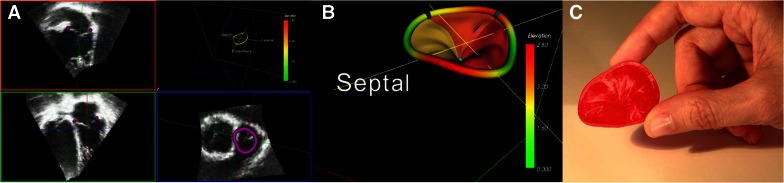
Three-dimensional printing of the tricuspid valve. From three-dimensional transthoracic echocardiographic data of the tricuspid valve, coordinates of the leaflets and annulus are demarcated (A) and imported to dedicated software to create the model (B) which is converted to stereolithographic file format and printed in 3D (C). Reproduced with permission from Muraru et al^6^
